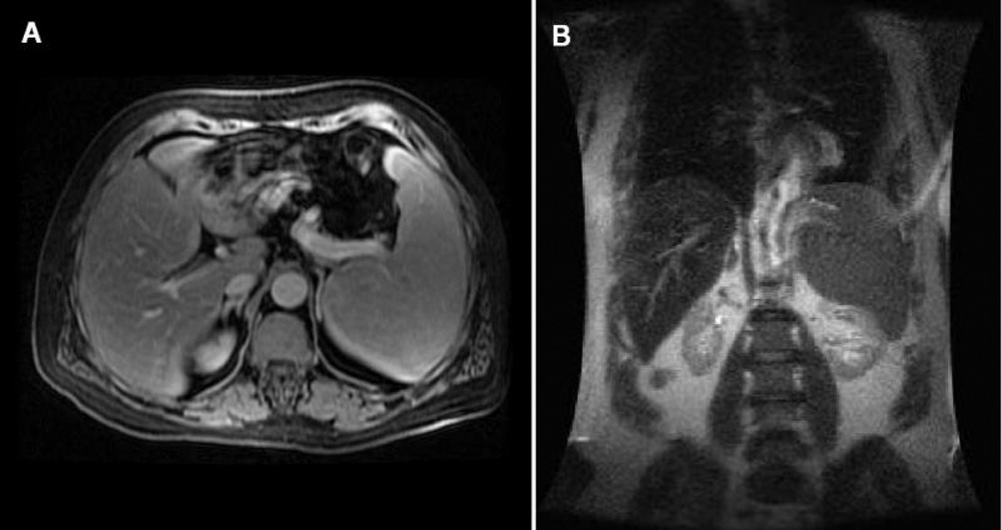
Axial (A) and coronal (B) view of the patient’s abdominal MRI. Splenomegaly is evident. Spleen size was calculated 18 cm × 15 cm × 11 cm. No focal lesions or hilar lymphadenopathy were detected.
